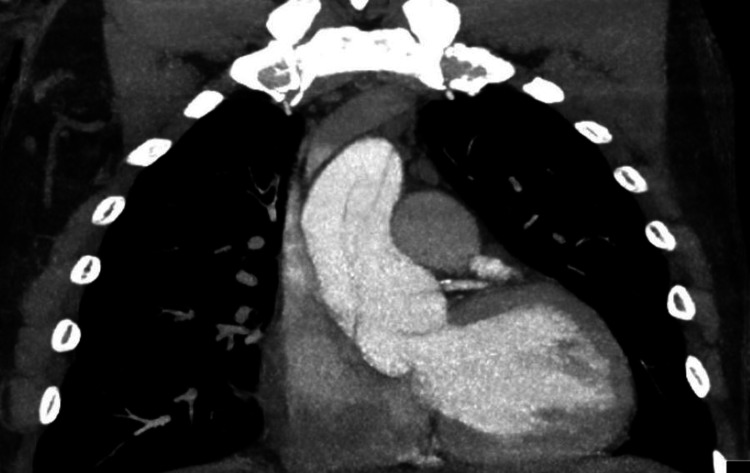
CT angiogram of the chest demonstrating aortic double lumen
